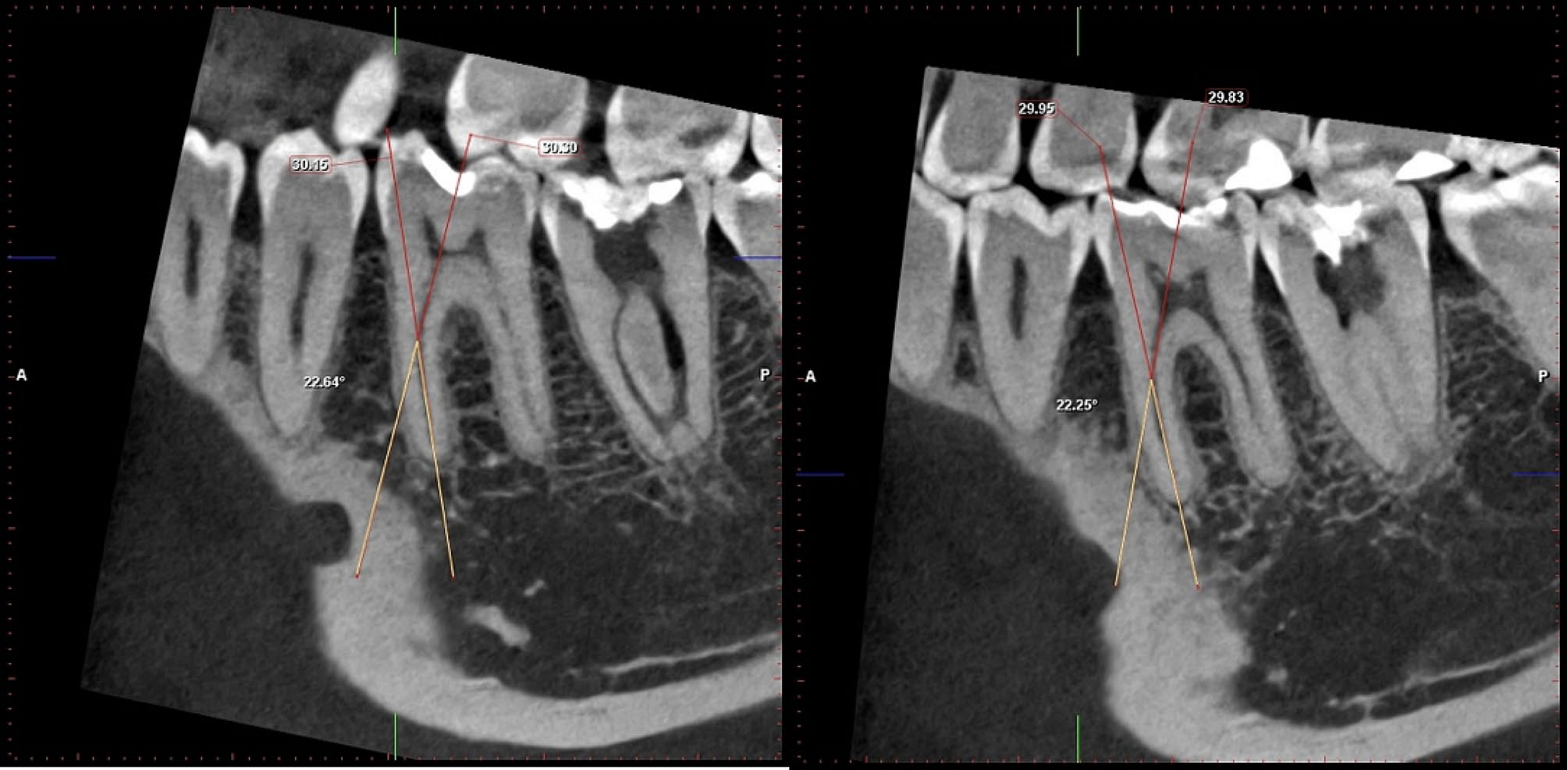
CBCT image showing measuring angle of curvature
